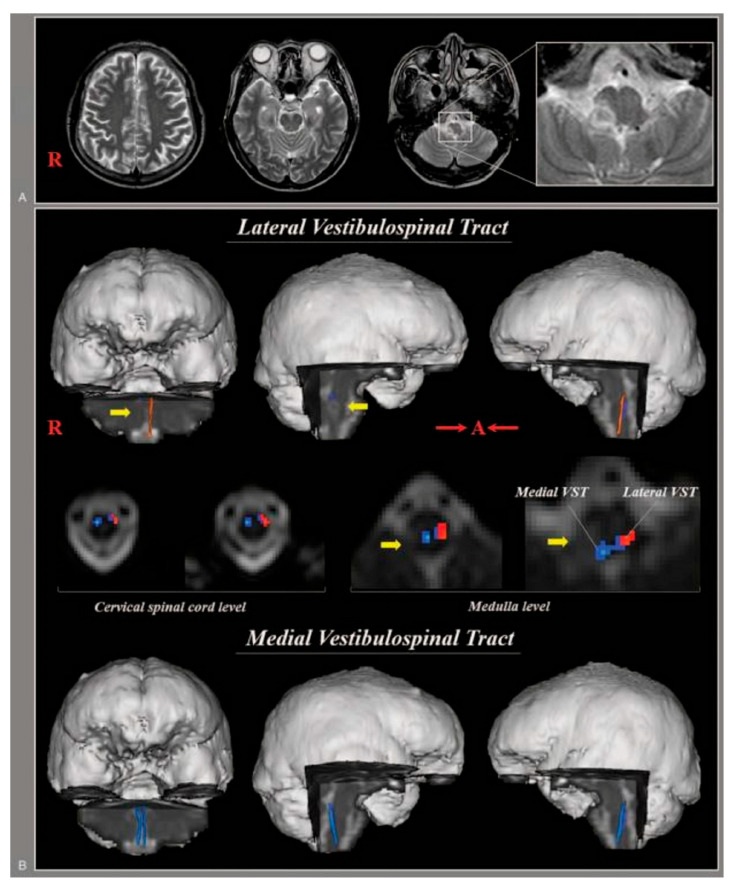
Anatomical structure of VST captured using DTT [38]. (A) Brain MR images at 2 weeks after onset show an infarction in the right dorsolateral medulla. (B) Results of diffusion tensor tractography (DTT). DTTs for both the medial vestibulospinal tract (VST) and the left lateral VST originate from the pontine vestibular nuclei and terminate at upper cervical cord. By contrast, DTT for the right lateral VST is not reconstructed between the lateral vestibular nuclei in pons and the upper cervical cord. Copyright by Jang et al. (2020) [38].
